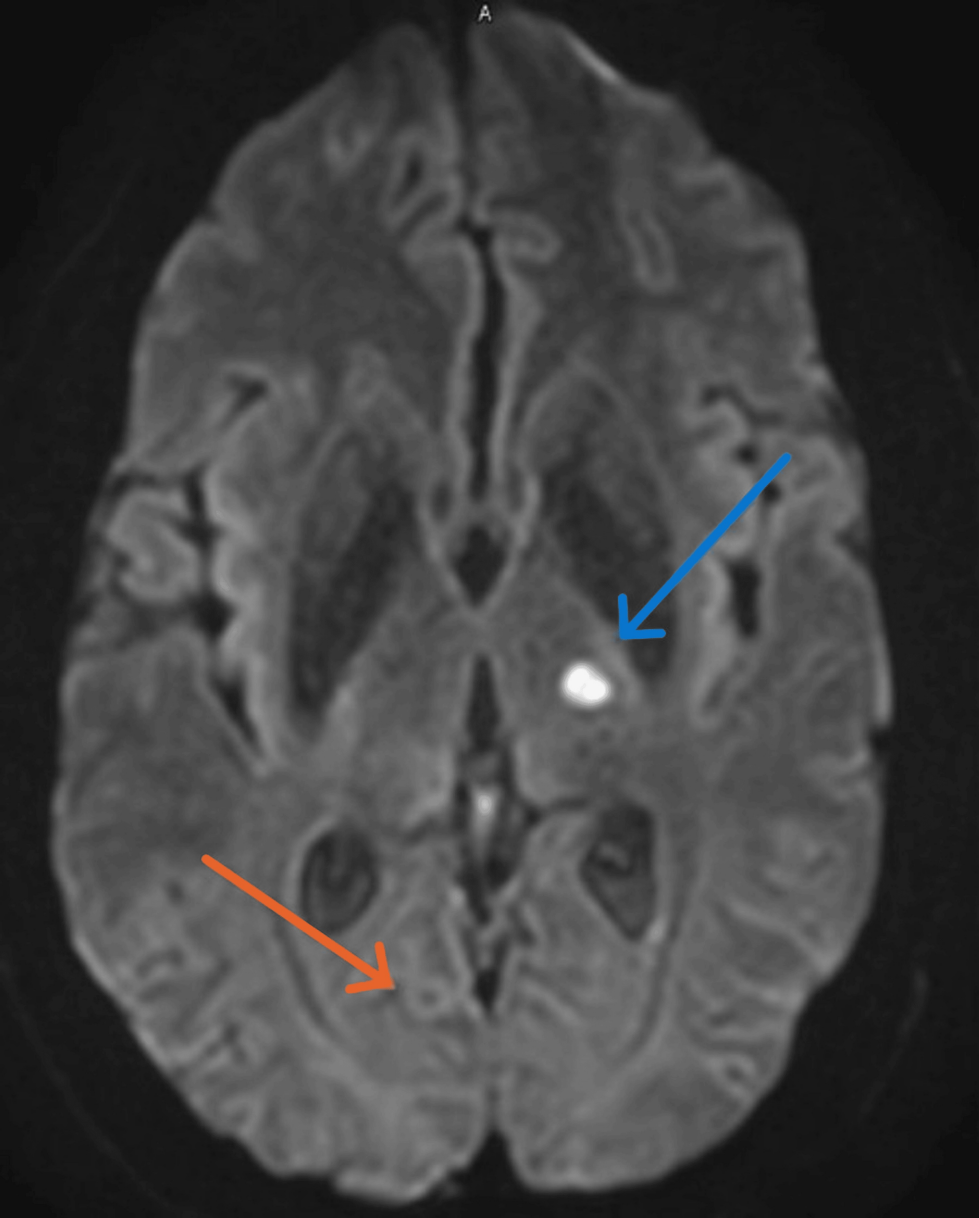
Head MRI shows left thalamus infracts (blue arrow), ring-enhancing lesion in the right occipital cortex exhibiting a central "dot sign," highly suggestive of neurocysticercosis (orange arrow).
